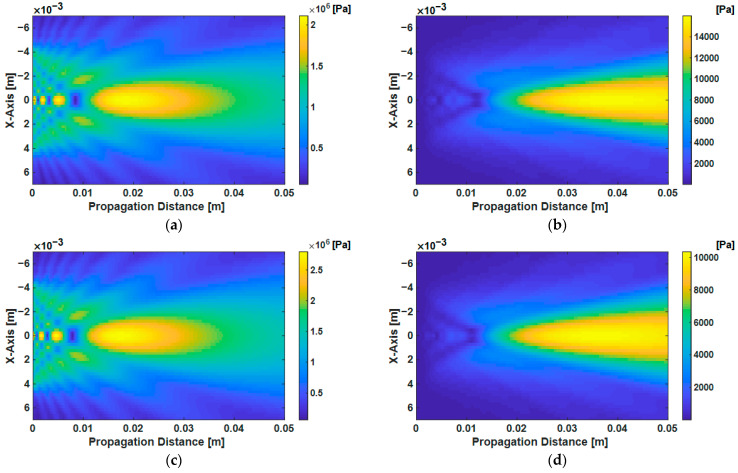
Numerical calculation results of the ultrasonic pressure field of fundamental wave $p_{1}\left(n\right)$ calculated using Equation (2), and second harmonic wave $p_{2_{material}}\left(n\right)$ using Equation (4) with = 0.1 m/s at 5 MHz driving frequency: (a) fundamental wave and (b) second harmonic wave in fused silica; (c) fundamental wave and (d) second harmonic wave in the Al6061-T6.
